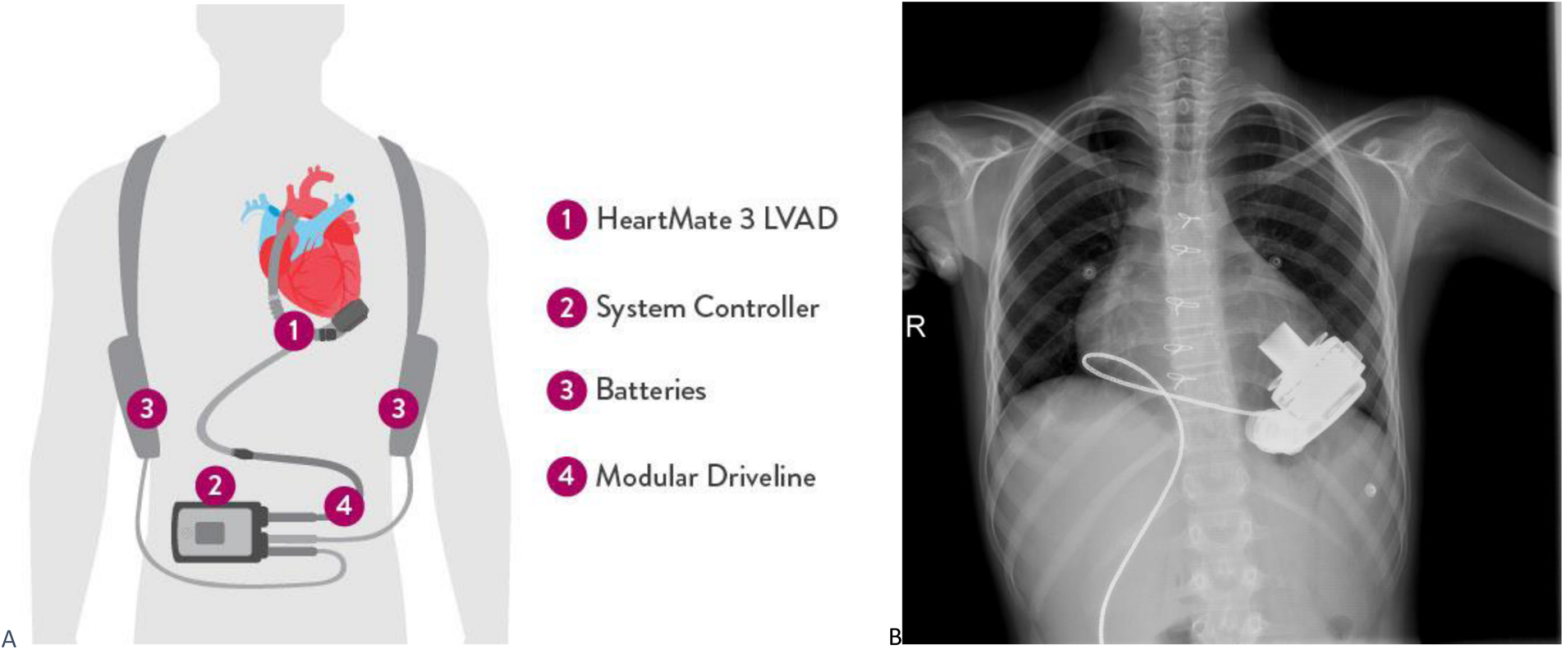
(A) Heartmate 3 device and equipments are shown. (B) PA chest radiograph of a pediatric patient implanted with HM3.
